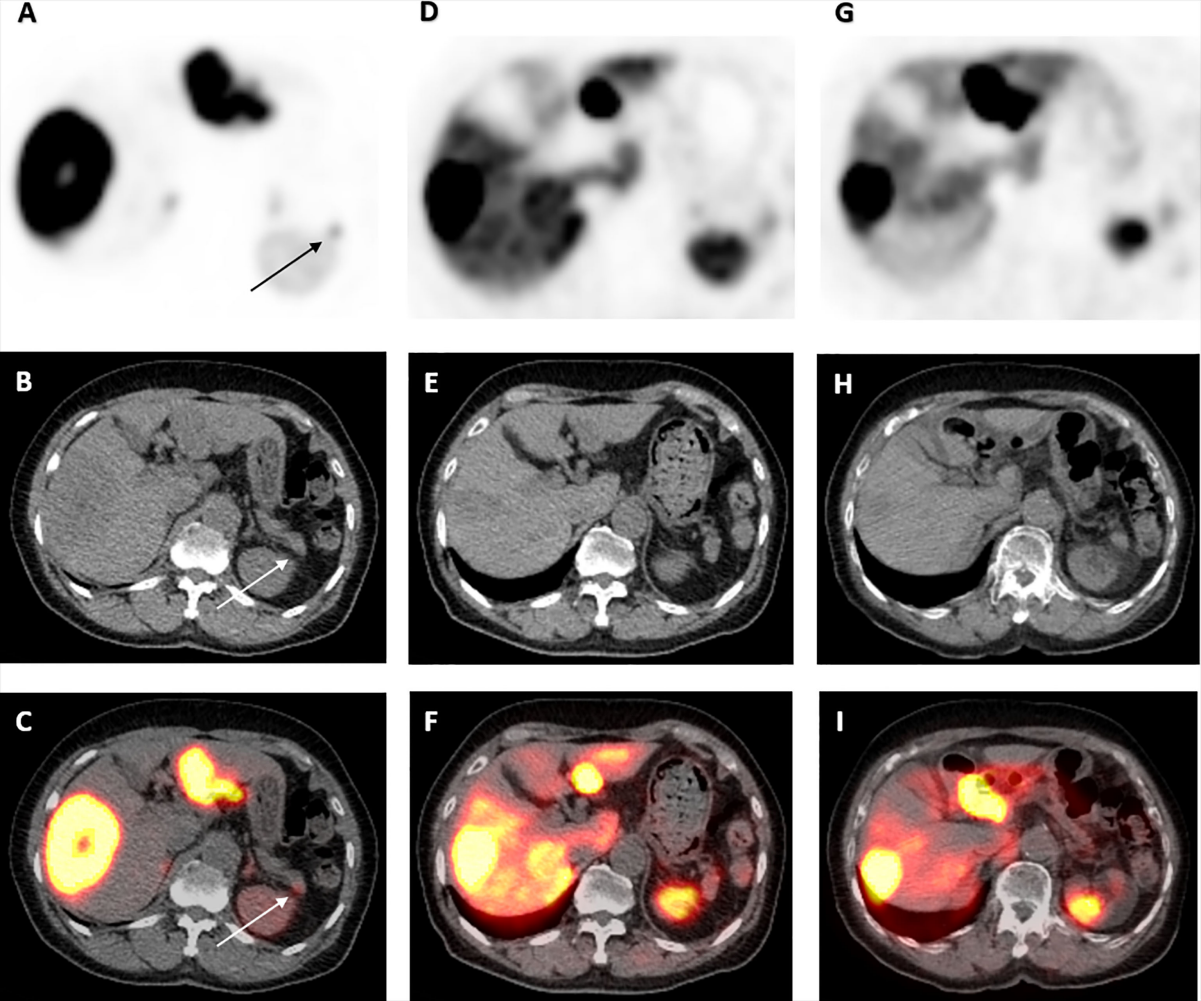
68Ga-DOTATATE PET/CT scan images of pancreatic tail lesion pre- and post-177 Lu-DOTATATE. Axial view images of ^68^Ga-DOTATATE PET/CT scan demonstrating interval reduction in avidity in moderately intense focus of activity at the tip of the pancreatic tail (arrow) on PET, low-dose CT and PET/CT fusion (top to bottom) from baseline, to 1 and 4 years after ^177^Lu-DOTATATE therapy (left to right). Physiological uptake in the spleen and remainder of the liver is also visualized.
